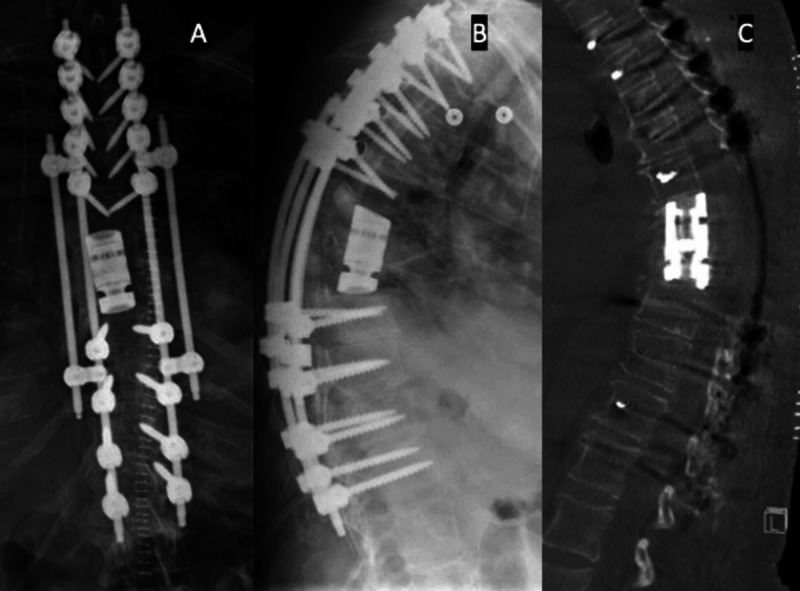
Post-operative imaging Postoperative imaging after the patient underwent vertebral column resection for the treatment of severe kyphotic deformity. A: anteroposterior X-ray. B: lateral X-ray showing T2-L1 instrumentation and intervertebral cage placement. C: sagittal CT scan reveals a significant reduction of kyphotic deformity.
